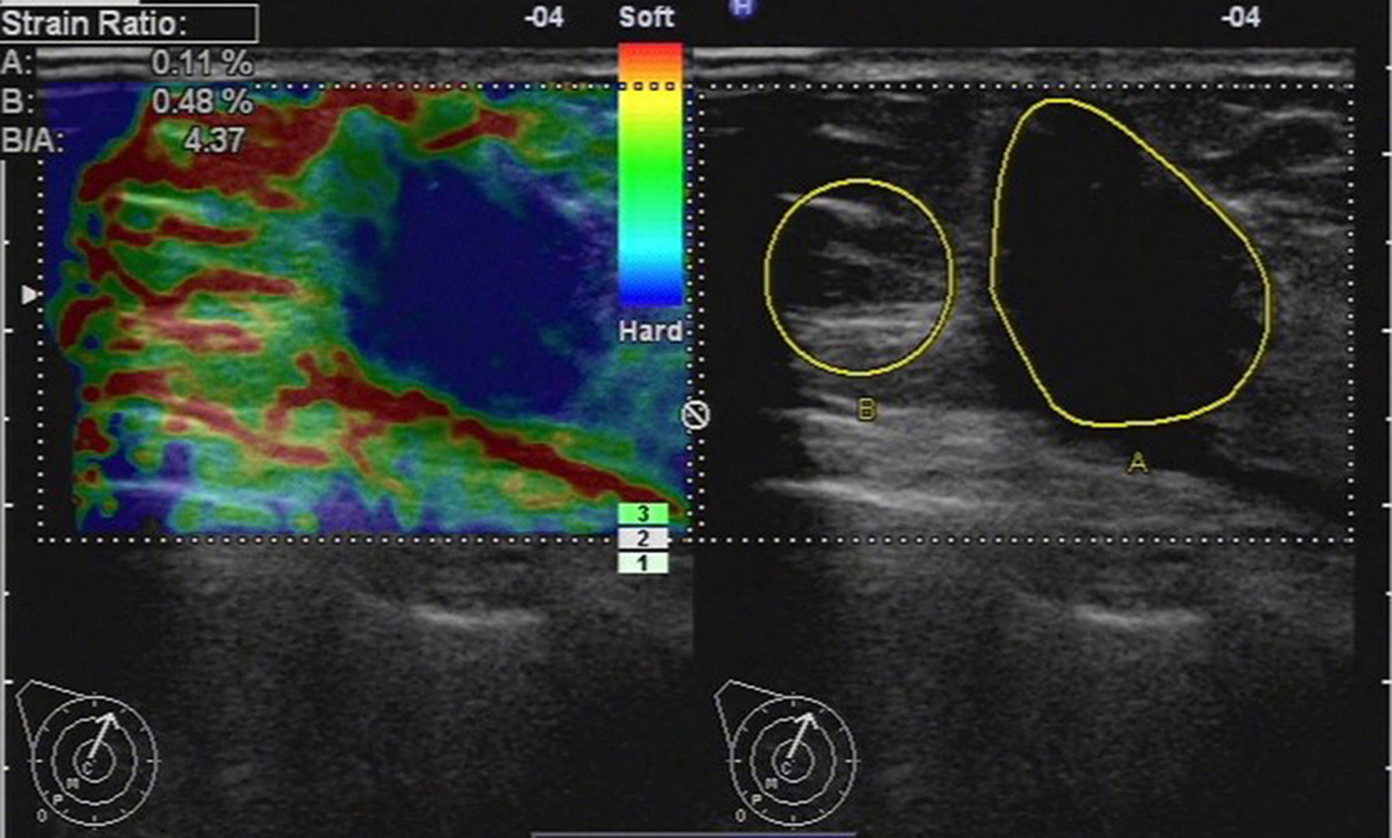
Ultrasonic elastographic image of a invasive lobular carcinoma in a 69-year-old woman. The lesion was scored 4 with the 5-point scoring method. The strain ratio was 4.37 with the strain ratio method at the same breast tissue depth as the reference.
